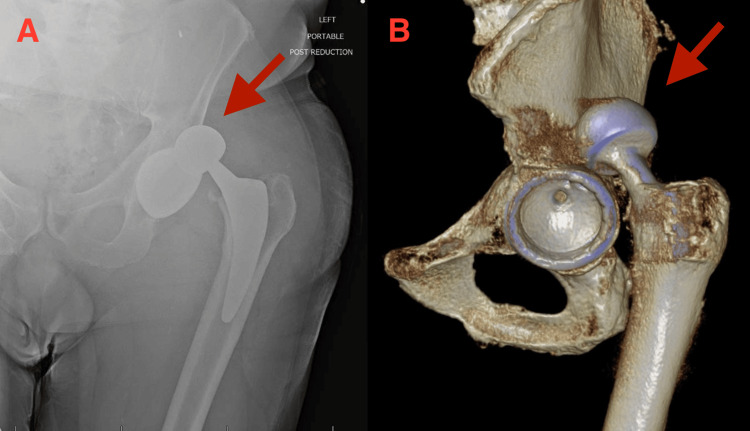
AP left hip radiograph (A) and three-dimensional CT scan view (B) demonstrating superior dislocation of left THA AP, anterior-posterior; THA, total hip arthroplasty; CT, computed tomography
